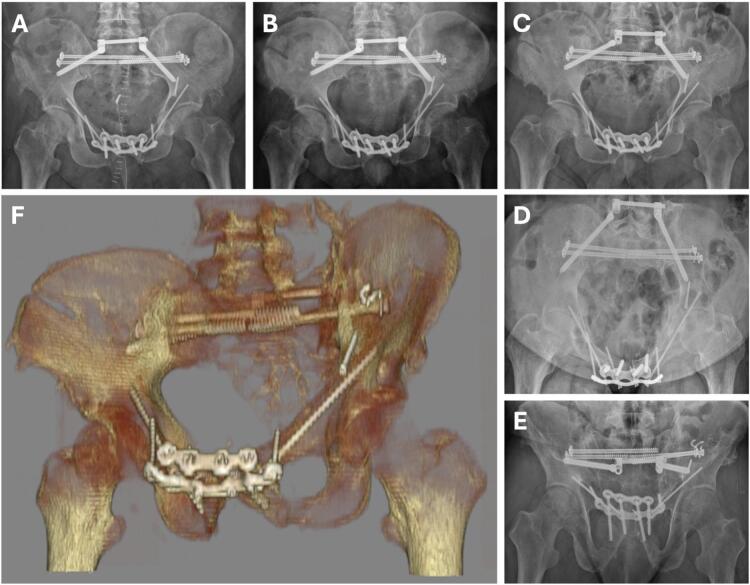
Anterior-posterior X-rays of the pelvis, postoperative after symphysiodesis with custom ultrathin plasma silver coated plates and screws (A), at 6-week follow-up (B), and at 5-year follow-up with loosening of the connector of the transiliac intern fixator including inlet and outlet views (C–E). 3D reconstruction of the final fixation (F).
